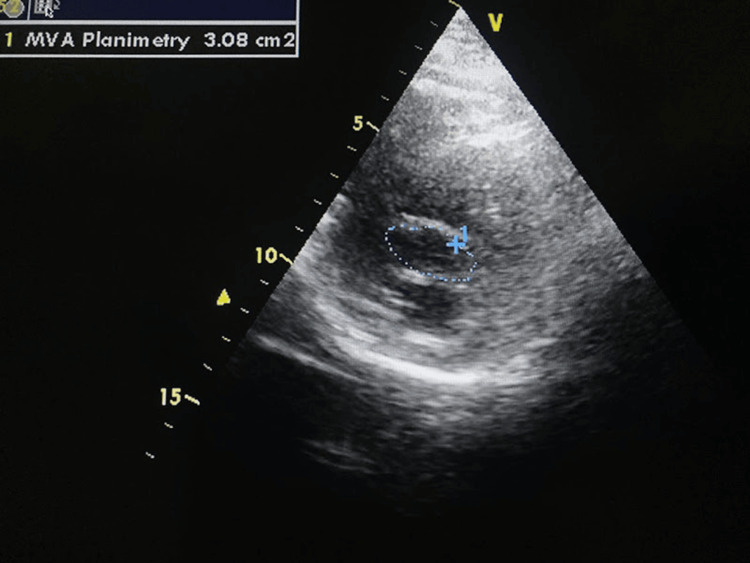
Transthoracic echocardiography (parasternal short-axis view) at the level of mitral valve leaflets demonstrating mitral orifice during diastole Manual tracing of mitral orifice shows a mitral valve area of 3.08 cm², which is within the normal range, indicating no residual mitral stenosis following open mitral commissurotomy.
